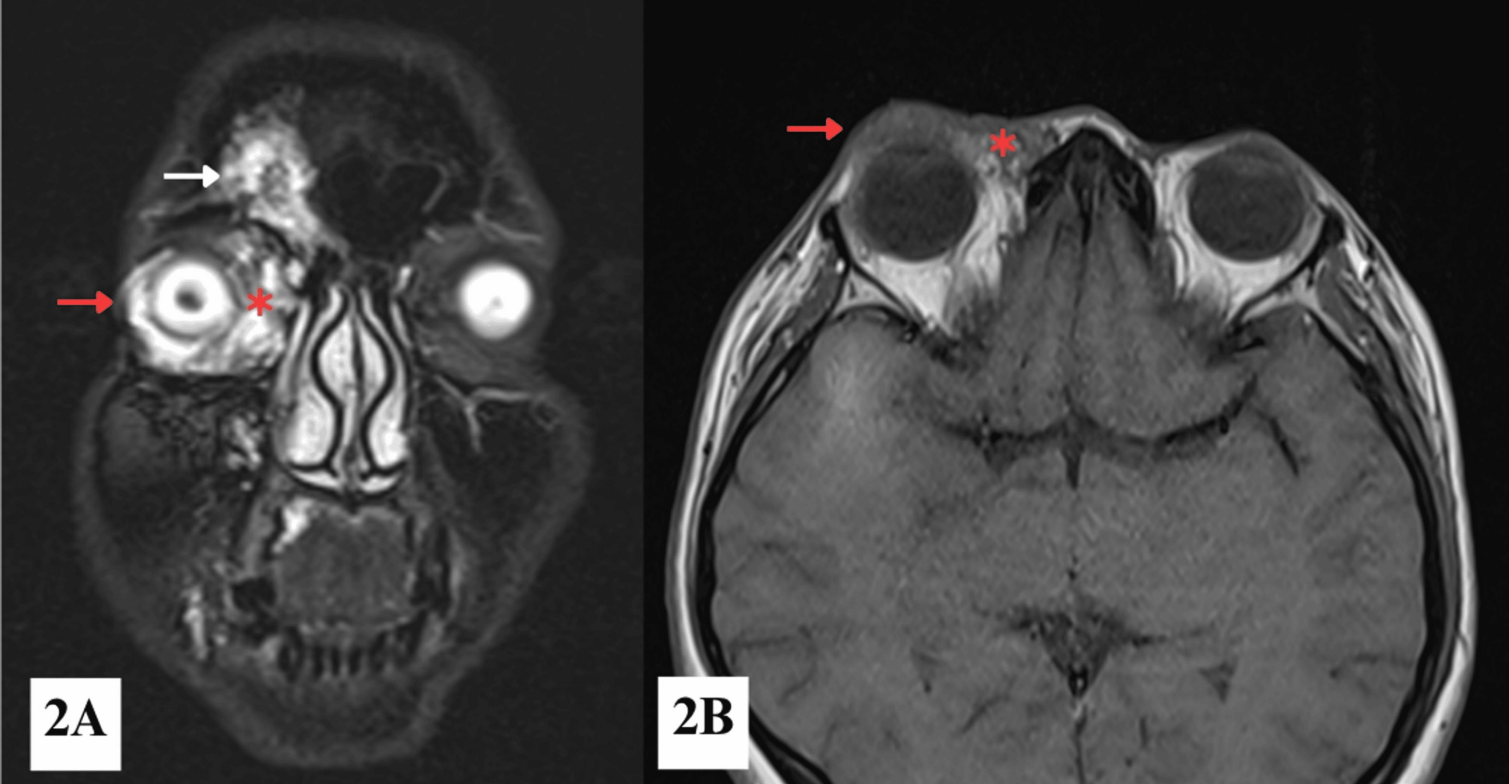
MRI showing (2A) a STIR coronal section of the face and (2B) a T1-weighted axial section of the orbit Multiple multilobulated lesions are seen involving the right frontal skull bone (white arrow), extending into the periorbital region and eyelid (red arrow), as well as the medial canthal area and the medial aspect of the right extraconal fat space (red asterisk).
